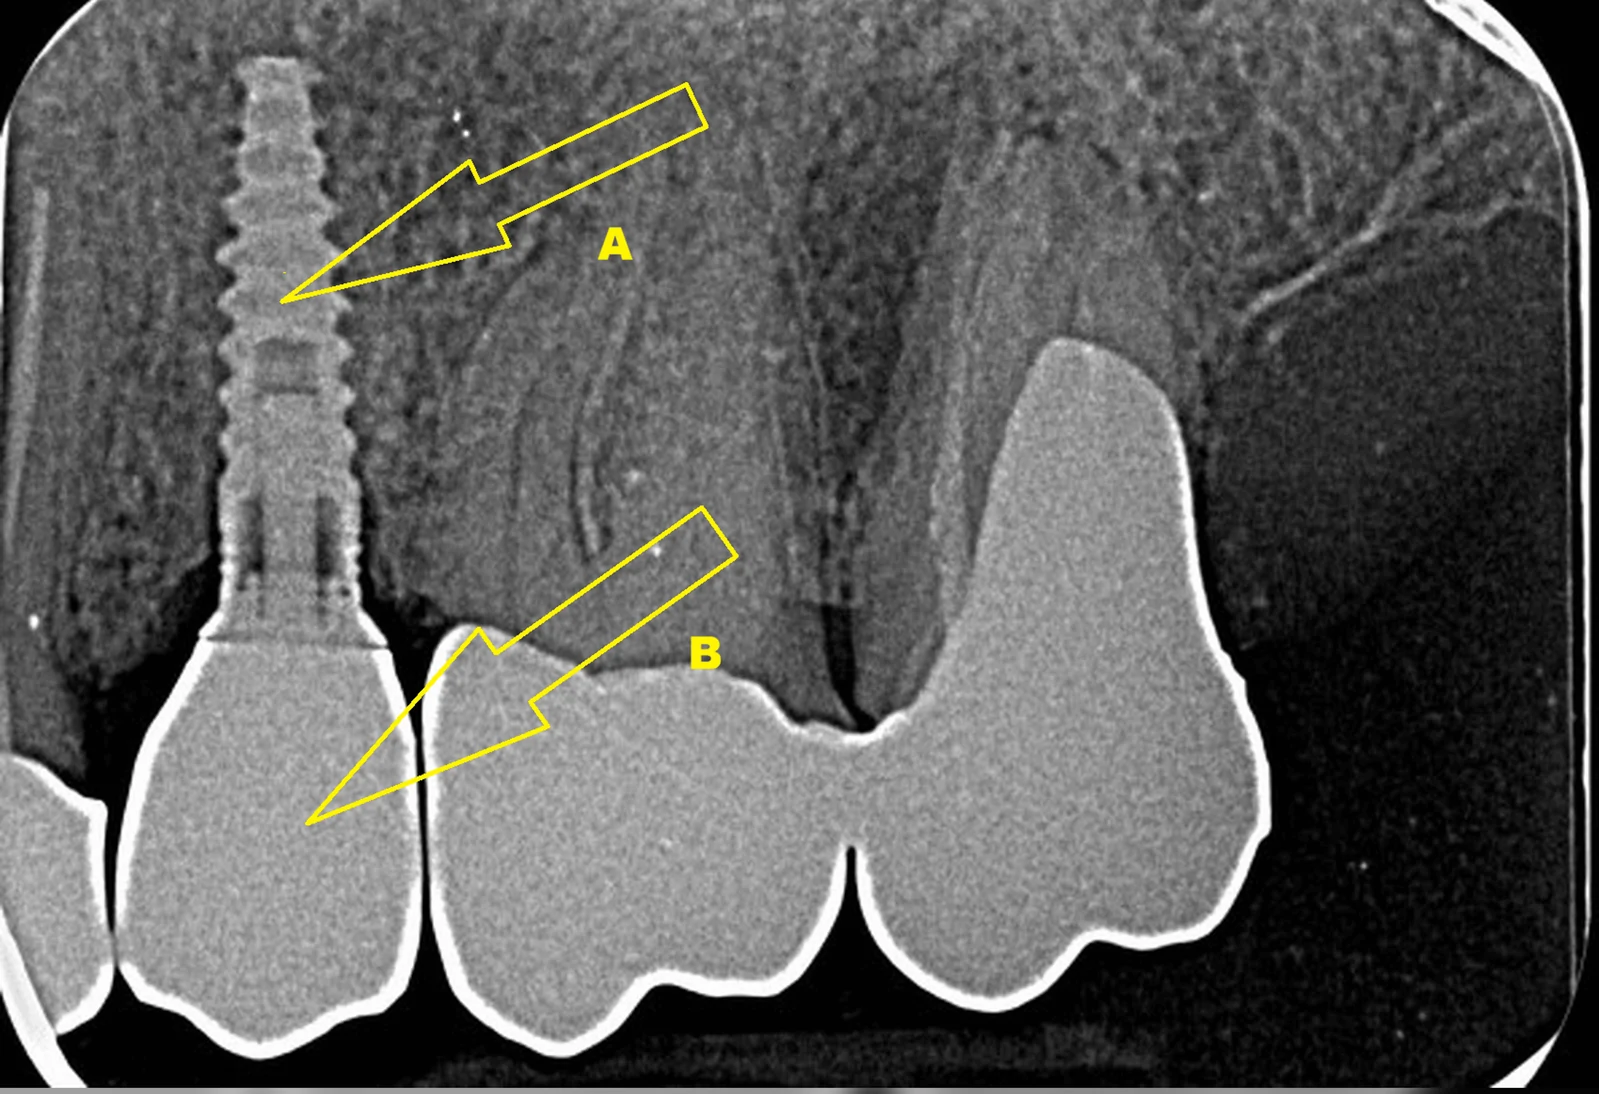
Periapical radiograph demonstrating the definitive implant-supported restoration of the maxillary right second premolar (tooth #4). Postoperative periapical radiograph obtained three months after implant placement, demonstrating successful osseointegration and delivery of the definitive implant-supported restoration replacing the maxillary right second premolar (tooth #4). Arrow A indicates the dental implant supporting the restoration. Arrow B indicates the definitive (permanent) implant-supported crown, demonstrating satisfactory adaptation and functional rehabilitation.
